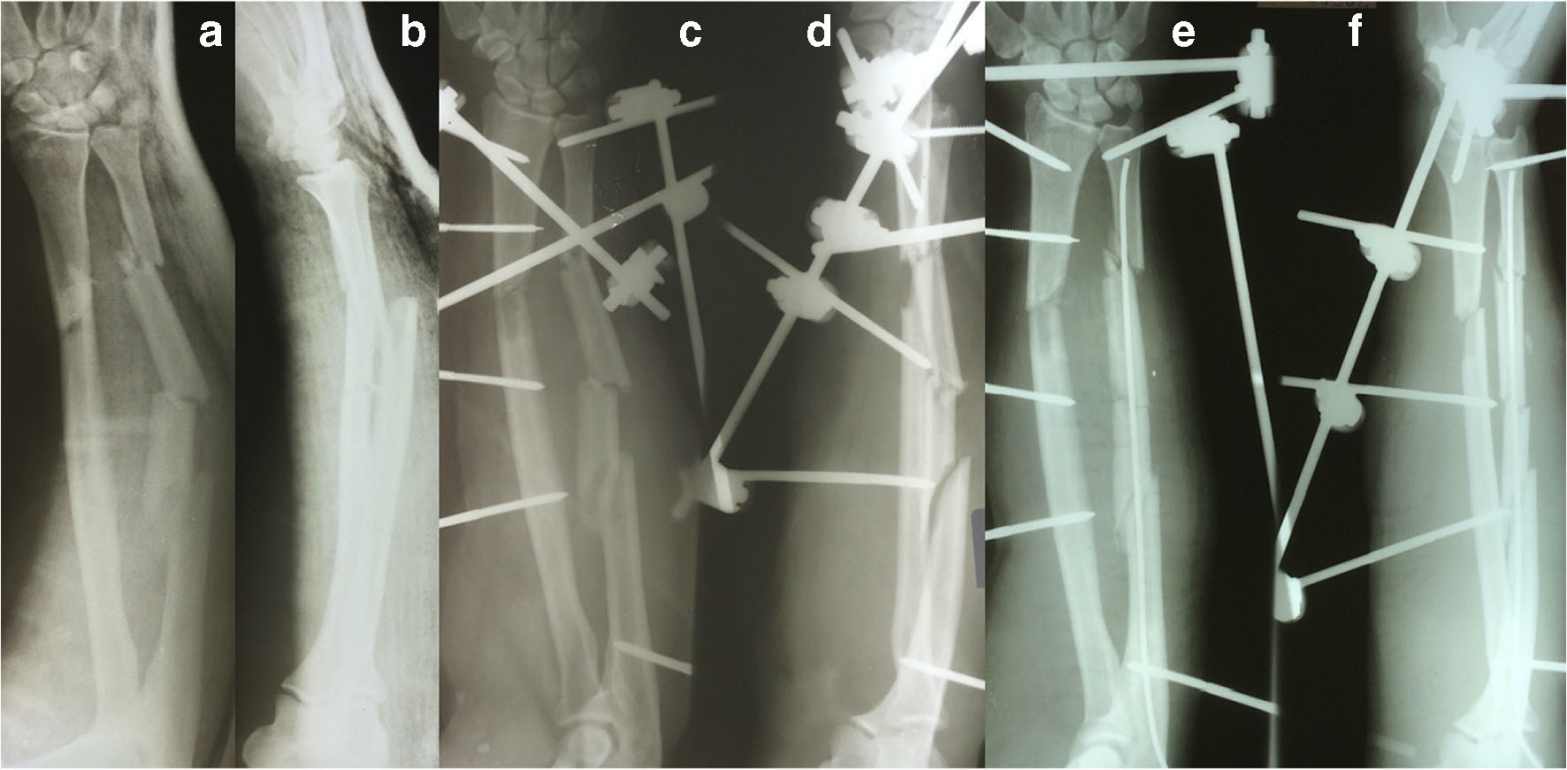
Anterior and lateral preoperative X-ray of the fractured forearm (a and b), III°B open of the patient mentioned in Fig. 2. Post-operative anteroposterior and lateral X-ray of the forearm showing the external fixator in place (c and d). Post-operative anteroposterior and lateral X-ray of the forearm showing the external fixator and an intramedullary K-wire to align the fracture (e and f). The K-wire was inserted at the last surgery before the skin was closed
